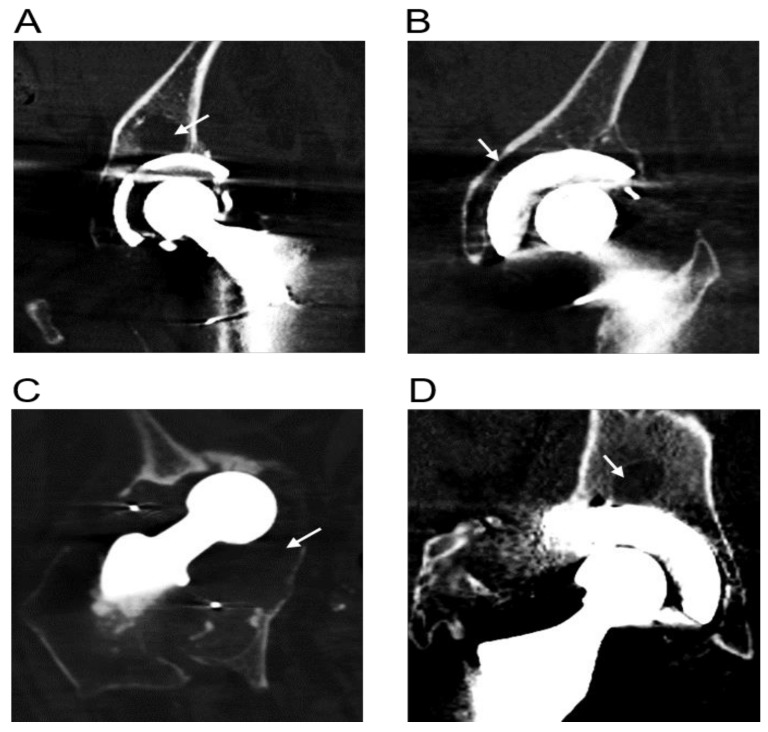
Representative radiographs of four revision patients with osteolytic lesions (white arrows). Corresponding patient and implant details are listed in Table 2 and are as follows: (A) Patient 1, (B) Patient 2, (C) Patient 8 and (D) Patient 14.
